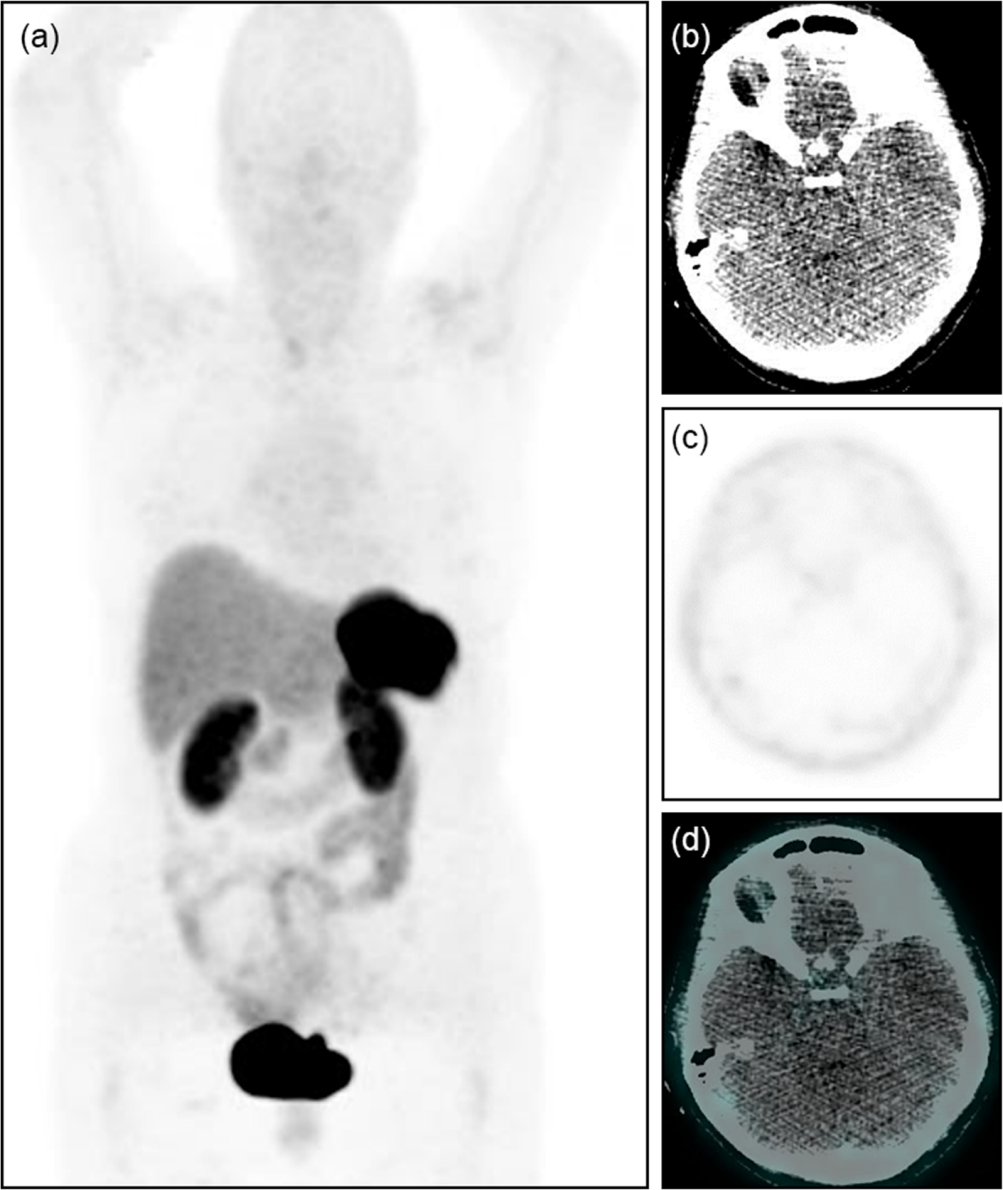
A 67-year-old woman history of surgical resection for ACTH pituitary macro-adenoma was referred with suspected recurrent Cushing disease. PET MIP (a), trans-axial CT, PET, and fused PET/CT images (b–d) show no [68Ga]Ga-DOTA-TATE uptake in pituitary fossa in keeping with prior curative resection. MRI showed no glandular tissue in pituitary fossa. The source of recurrent ACTH in this case was unknown
